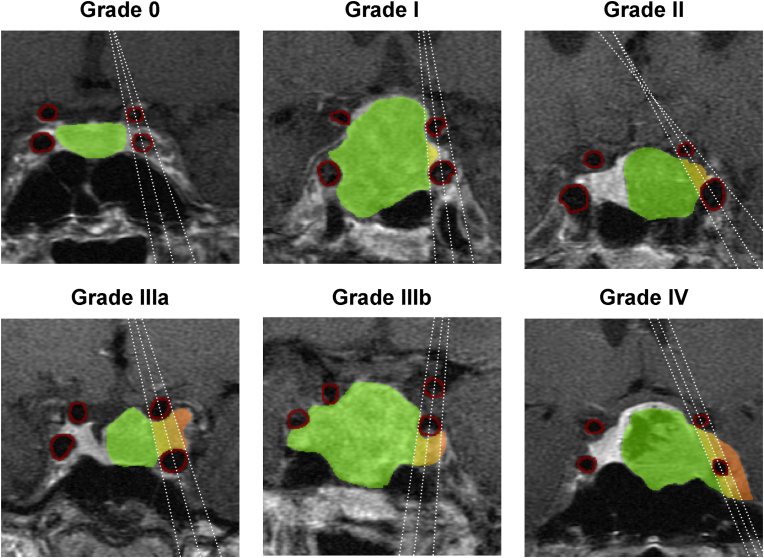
Magnetic Resonance Imaging (MRI) scans demonstrating the Knosp classification of pituitary adenomas, with varying degrees of tumor extension and invasion into the cavernous sinus. The scans are segmented to highlight the tumor in green and the internal carotid artery in red. The progression of grades from 0 to IV reflects the increasing level of tumor invasion, with Grades IIIa and IIIb indicating different patterns of growth in relation to the carotid artery. The medial tangent, connecting line of the centers and the lateral tangent are displayed for the ICA cross-sections on the right side.
